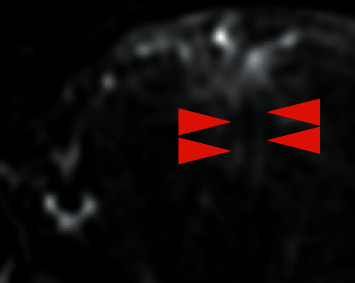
Pelvic MRI of endometrial stromal sarcoma: in DCE-MRI, angiogram depicted tumor regions containing dense wide, regular, and parallel vessels (arrowheads).
